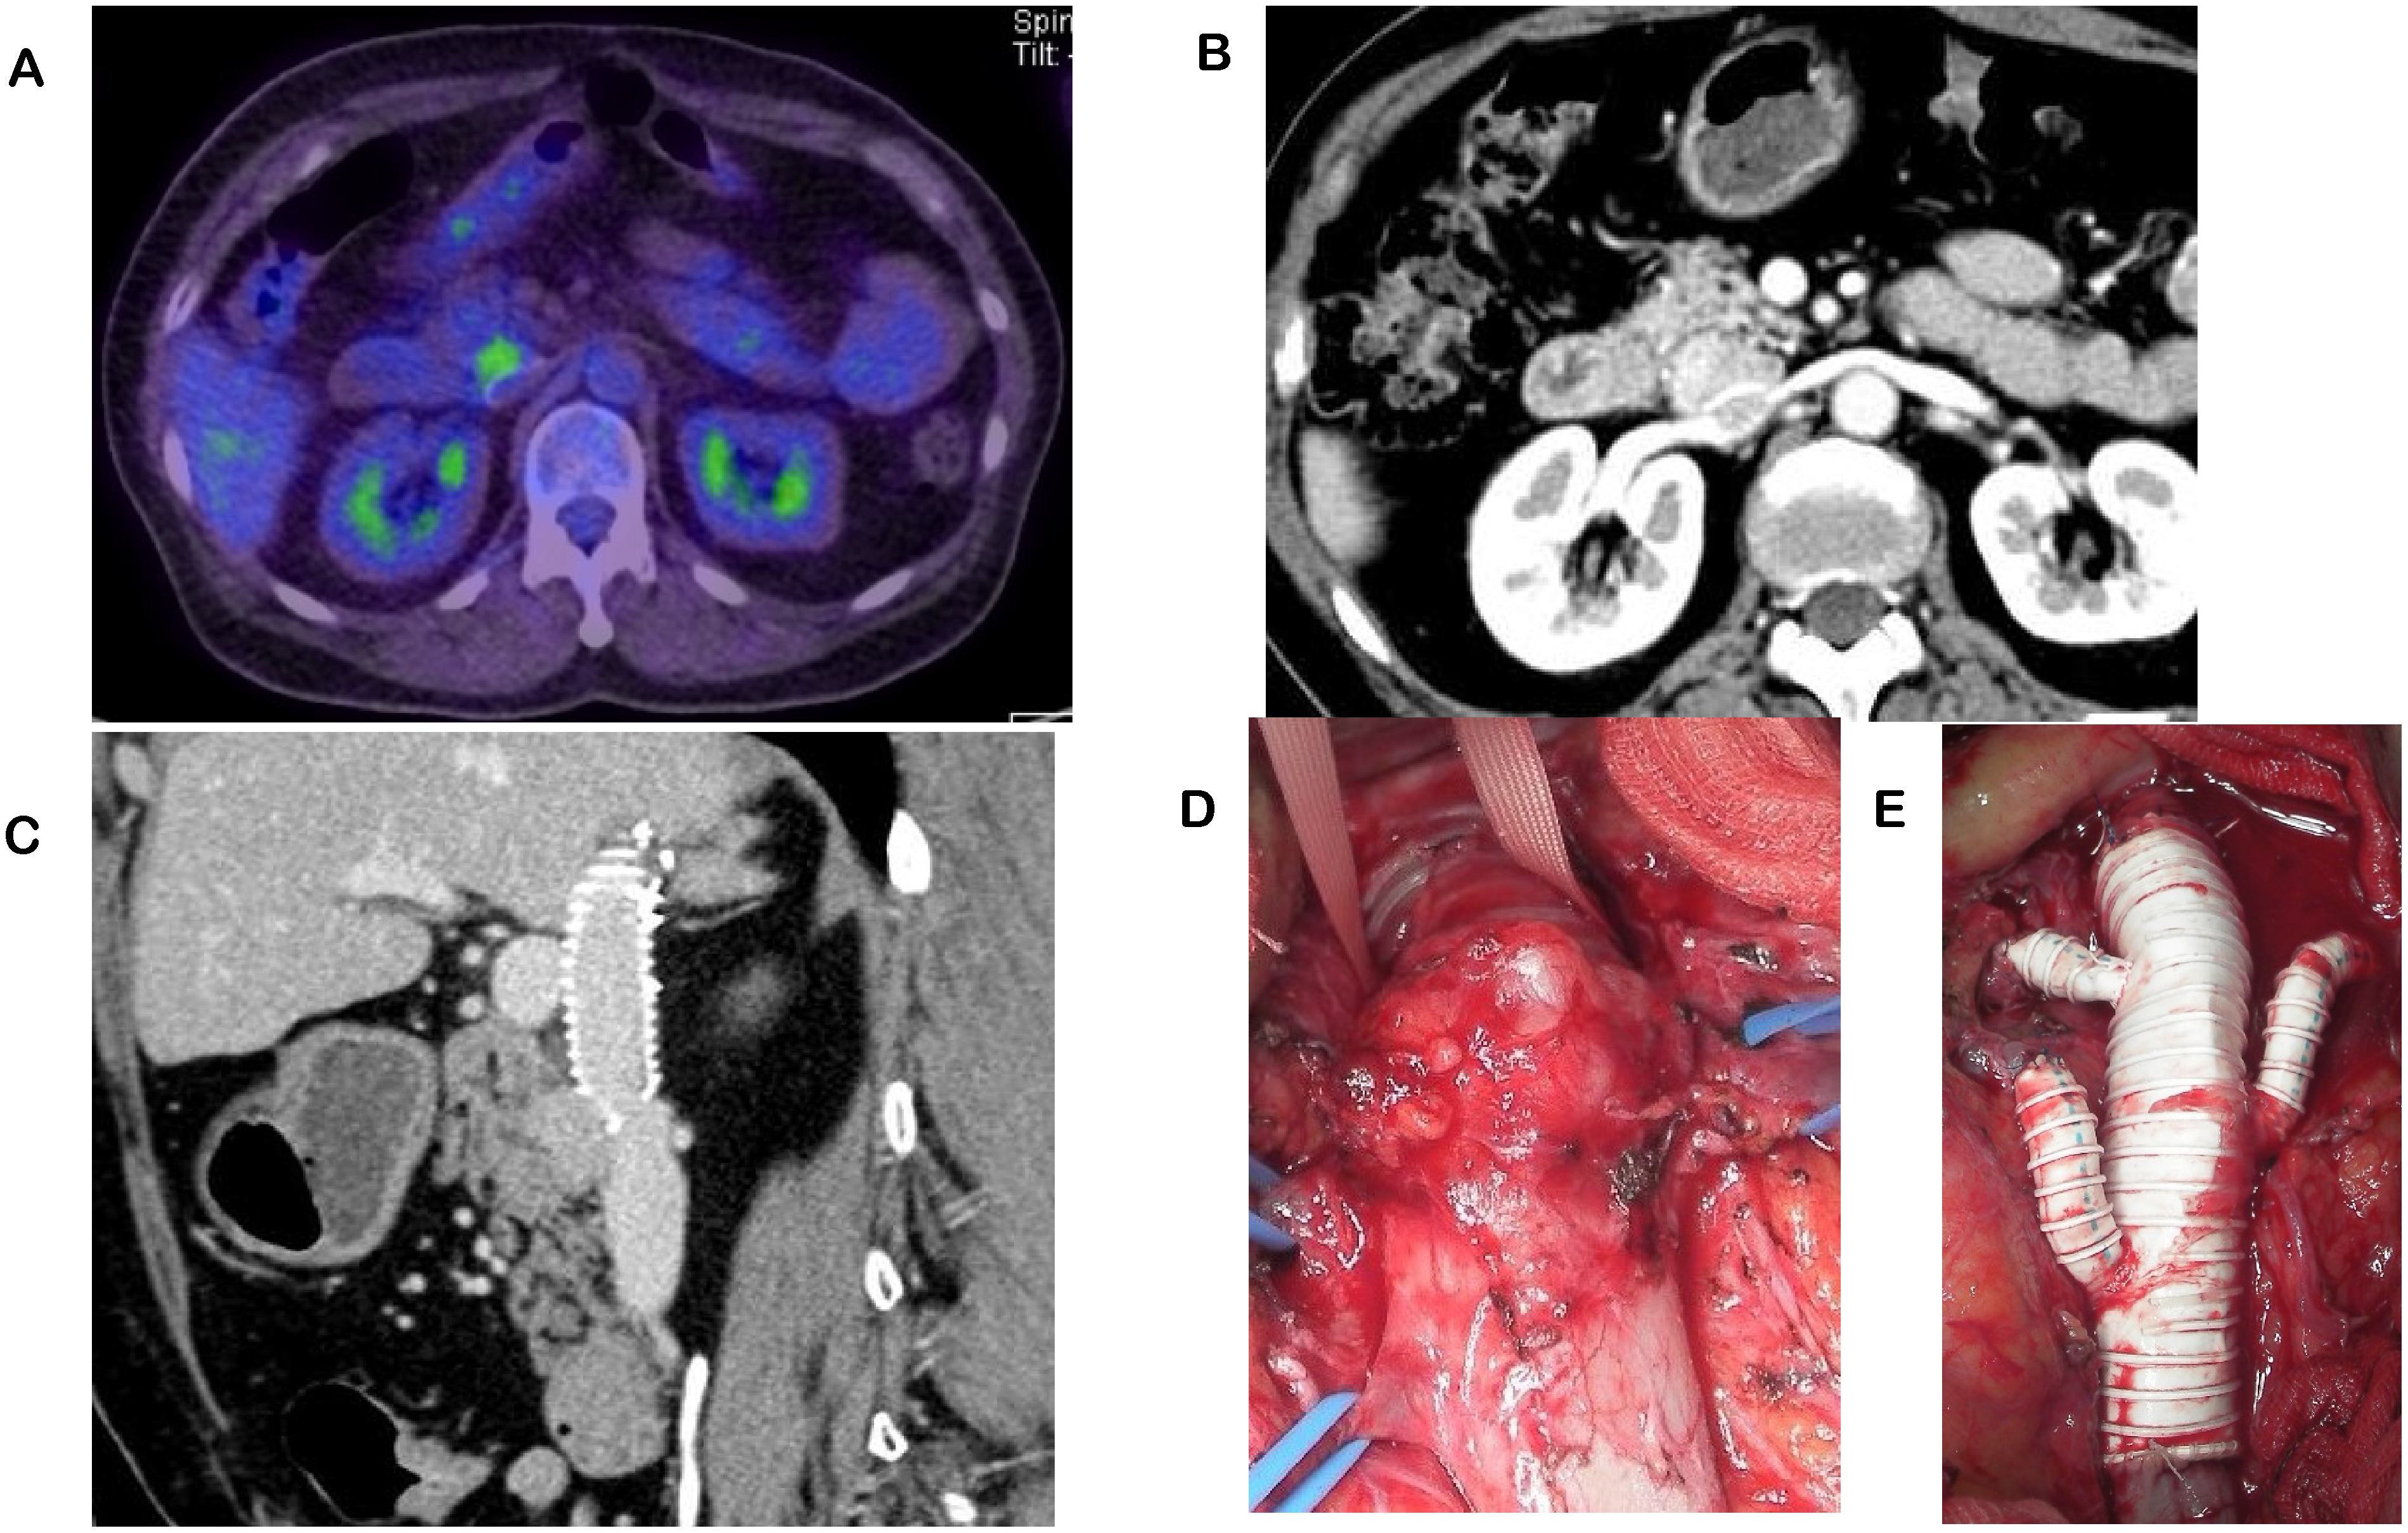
Fig. 2 (A) Positron emission tomography (PET)-computed tomography (CT) 2 years after the first operation shows an intense accumulation of fluorodeoxyglucose (FDG) at the anastomosis on the distal side of the graft. (B) Contrast-enhanced CT reveals an extramural mass approximately 2 cm in diameter growing on the lower side of the head of the pancreas. (C) The tumor is present on the front of the site of anastomosis between the graft and inferior vena cava (IVC). (D) The intraoperative image shows the tumor has grown to outside the IVC. The capsule is strong, and the pancreas and neighboring organs show no macroscopic infiltration. (E) The IVC is replaced, and the renal veins (double right renal veins and single left vein) are reconstructed using a branched graft.
